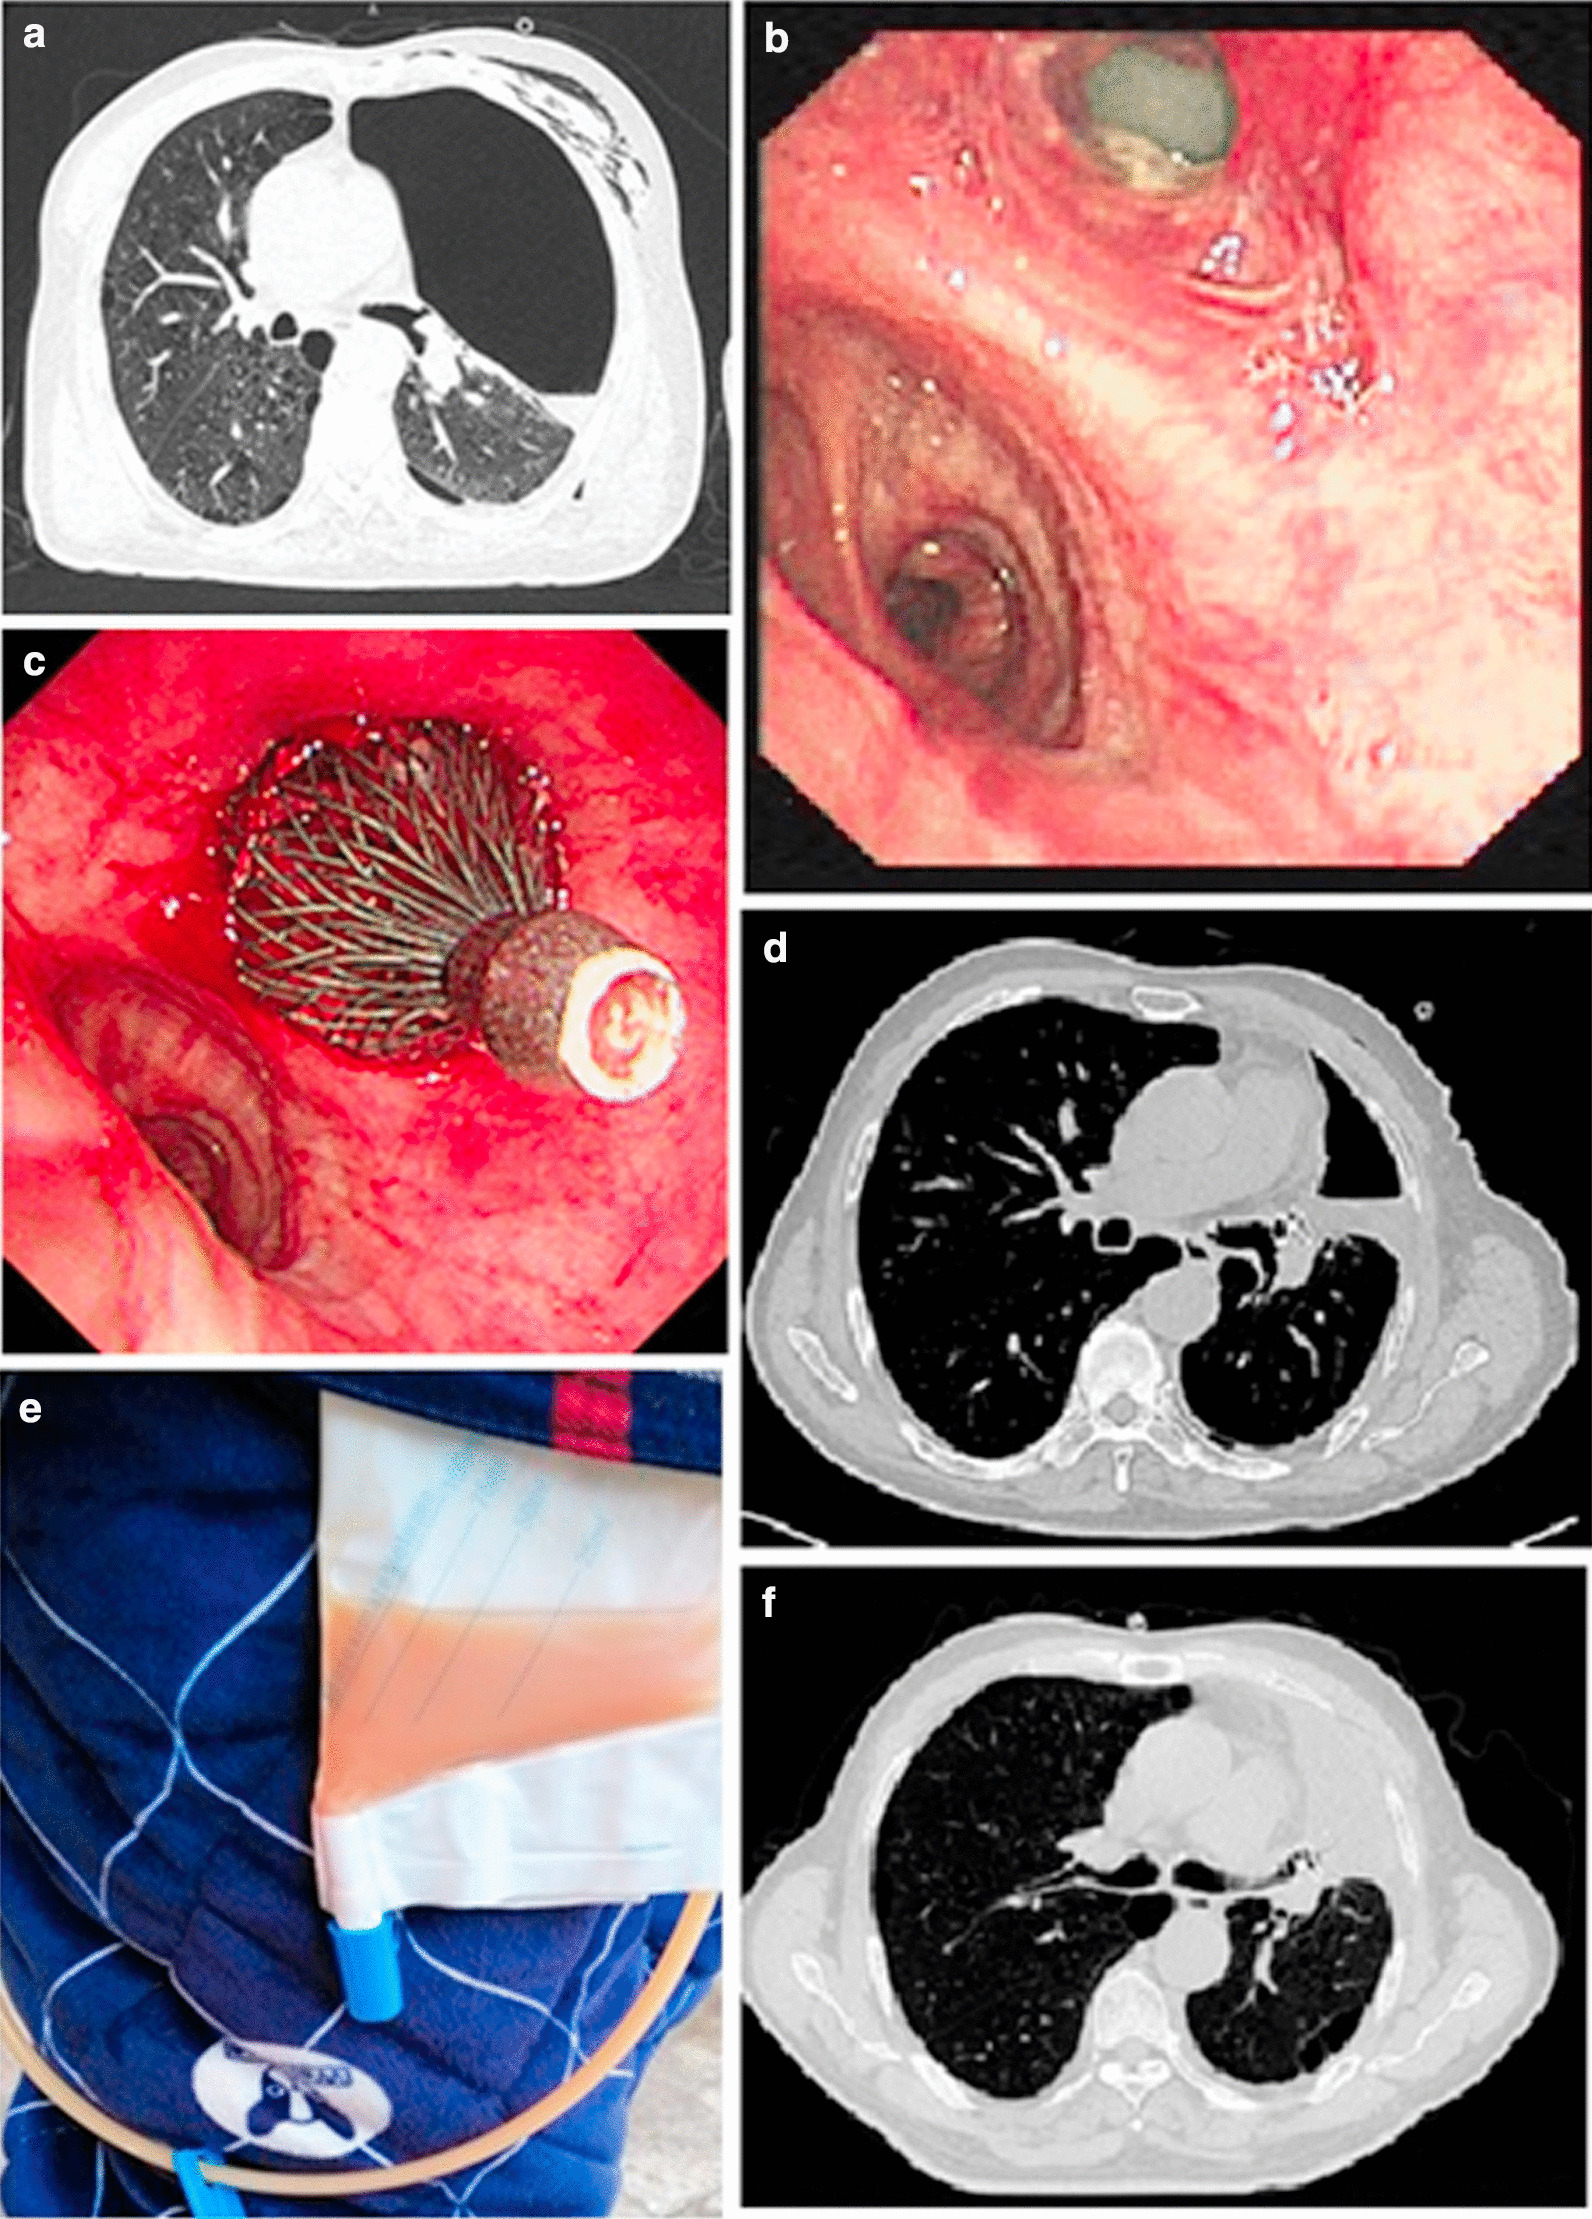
Bronchopleural fistula following left upper lobe lobectomy in patient No. 4. a Chest CT before AD implantation. b Bronchoscopy before AD implantation. c Bronchoscopy after AD implantation. d Chest CT after 1 month of AD implantation. e Water seal drainage bottle was changed to a drainage pack after 1 month of AD implantation. f Chest CT after 2 months of AD implantation, and the drainage tube was removed after CT scan
